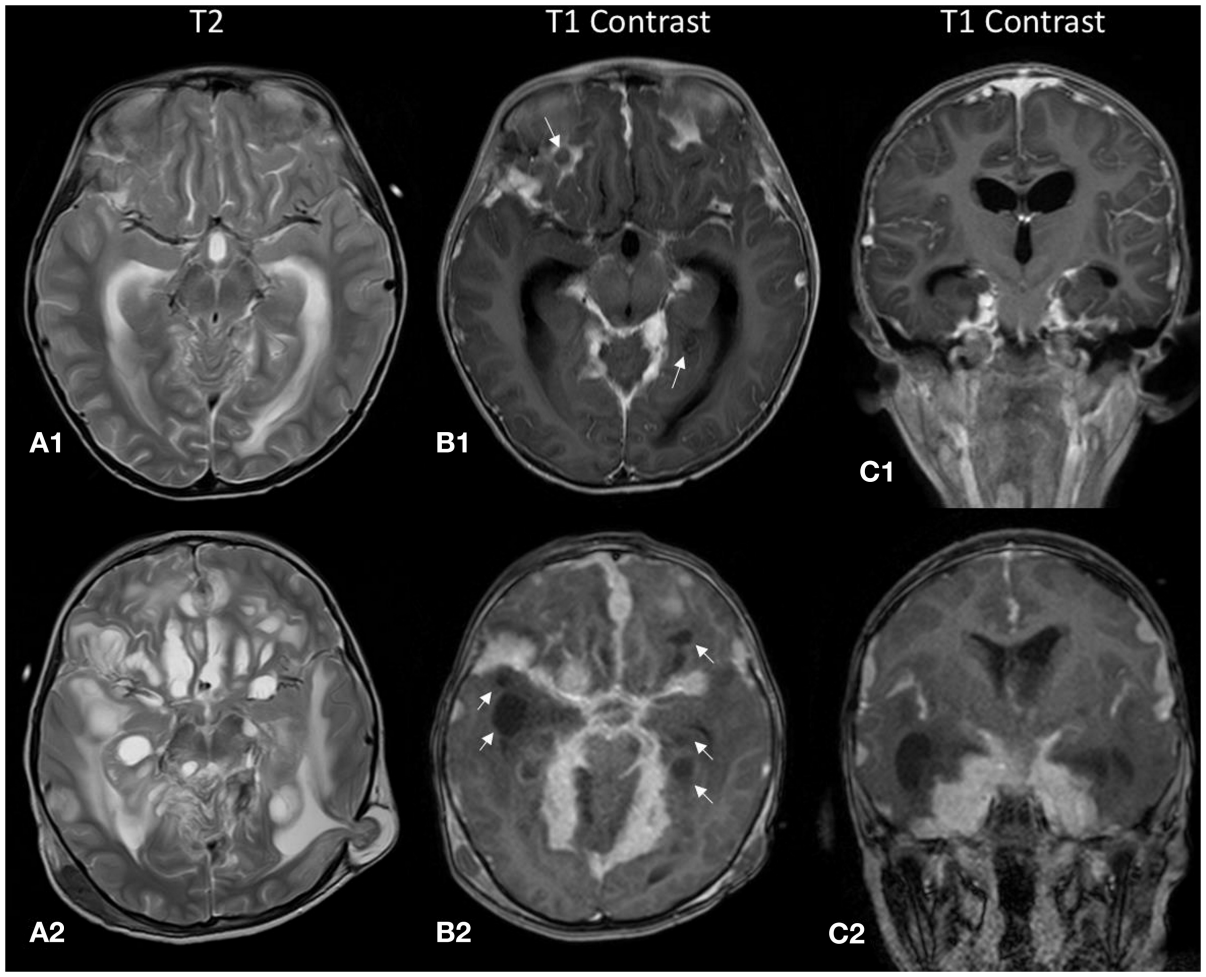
The top row (A1–C1) displays MR images from the initial study during the first admission, and the bottom row (A2–C2) displays the corresponding images during the relapse 2 months later. (A1,A2) There were cystic lesions with high T2 signal along the leptomeningeal surface. These initially small non-enhancing cystic lesions [white arrows, (B1)] increased in size and number in the follow-up scan [white arrows, (B2)]. (A2) Brain tissue was seen herniating through a Burr hole from a previous external ventricular shunt site, indicating that there was increased intracranial pressure. (C1,C2) Coronal post-contrast T1 images demonstrate the predominantly basal cisternal location of the nodular leptomeningeal thickening.
